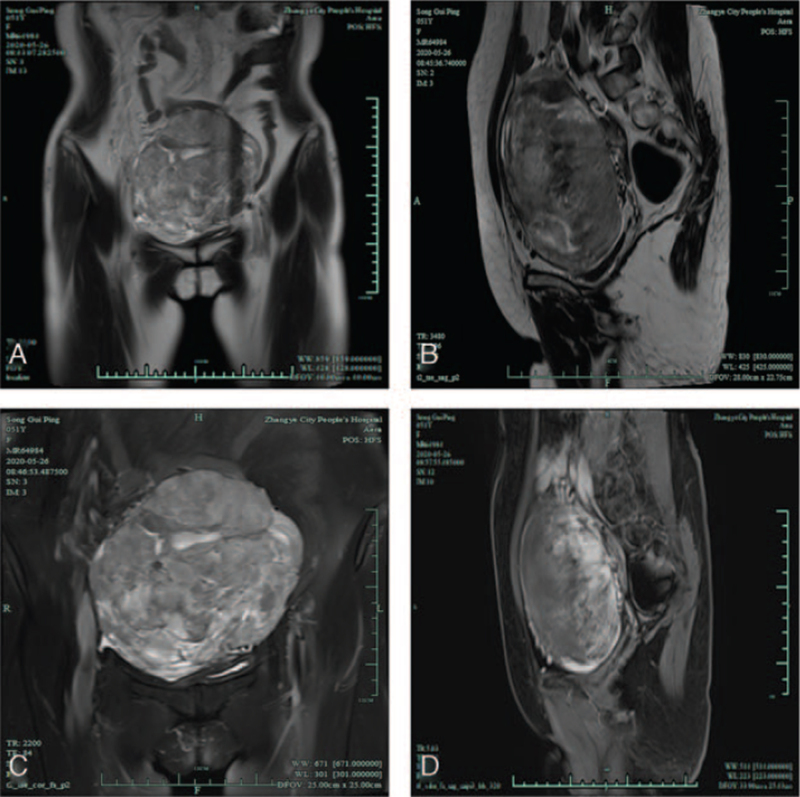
Magnetic resonance imaging of the pelvic cavity mass: (A) Coronal section; (B) Sagittal section; (C) Contrast-enhanced image of the coronal section; (D) Contrast-enhanced image of the sagittal section.
